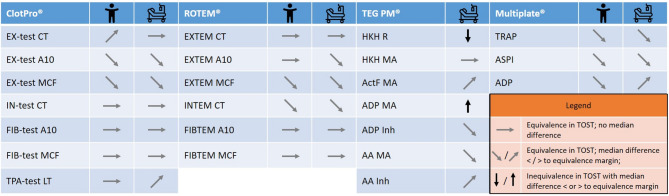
Practical summary of transport effects on viscoelastic and platelet function assays. Paired analyses after manual versus pneumatic tube transport across all analyzers (ClotPro, ROTEM, TEG PlateletMapping, and Multiplate). Each field represents one assay parameter (e.g. CT, A10, MCF, MA, LT). The left column shows results for healthy volunteers, the right column for critically ill patients with sepsis. Color coding denotes equivalence testing (TOST): - Grey: Equivalent results in TOST – observed difference remains within expected intra-device variability; - Black: Not equivalent in TOST – results require cautious interpretation when pneumatic tube transport is used. Arrow direction indicates whether the median difference lies below or above the manufacturer-reported coefficient of variation (CV), reflecting the direction and relative magnitude of deviation.
